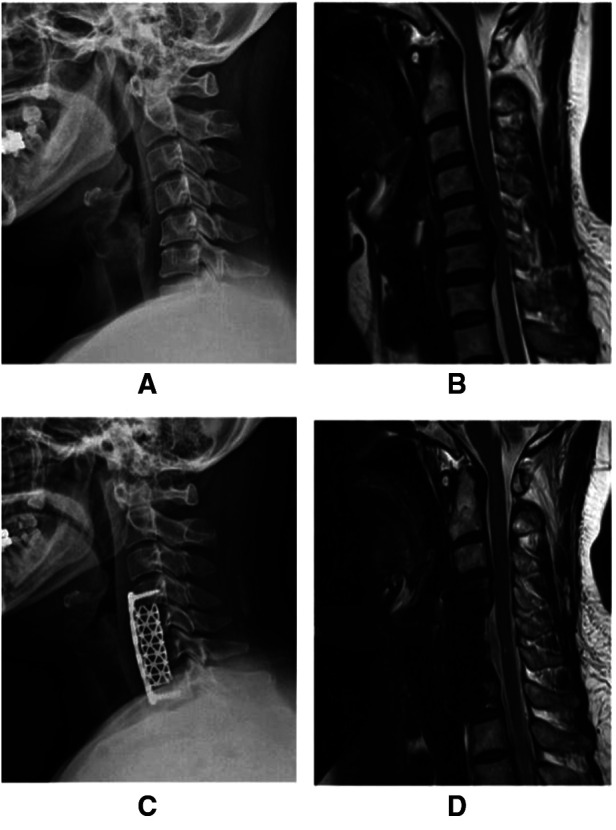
Anterior cervical subtotal corpectomy with titanium plate and screw fixation. (A) Lateral radiograph showing cervical kyphosis. (B) Posterior compressing of the spinal cord at the C4/5–C6/7 level. (C) Postoperative improved cervical alignment. (D) Postoperative MRI showing the decompressed spinal cord.
